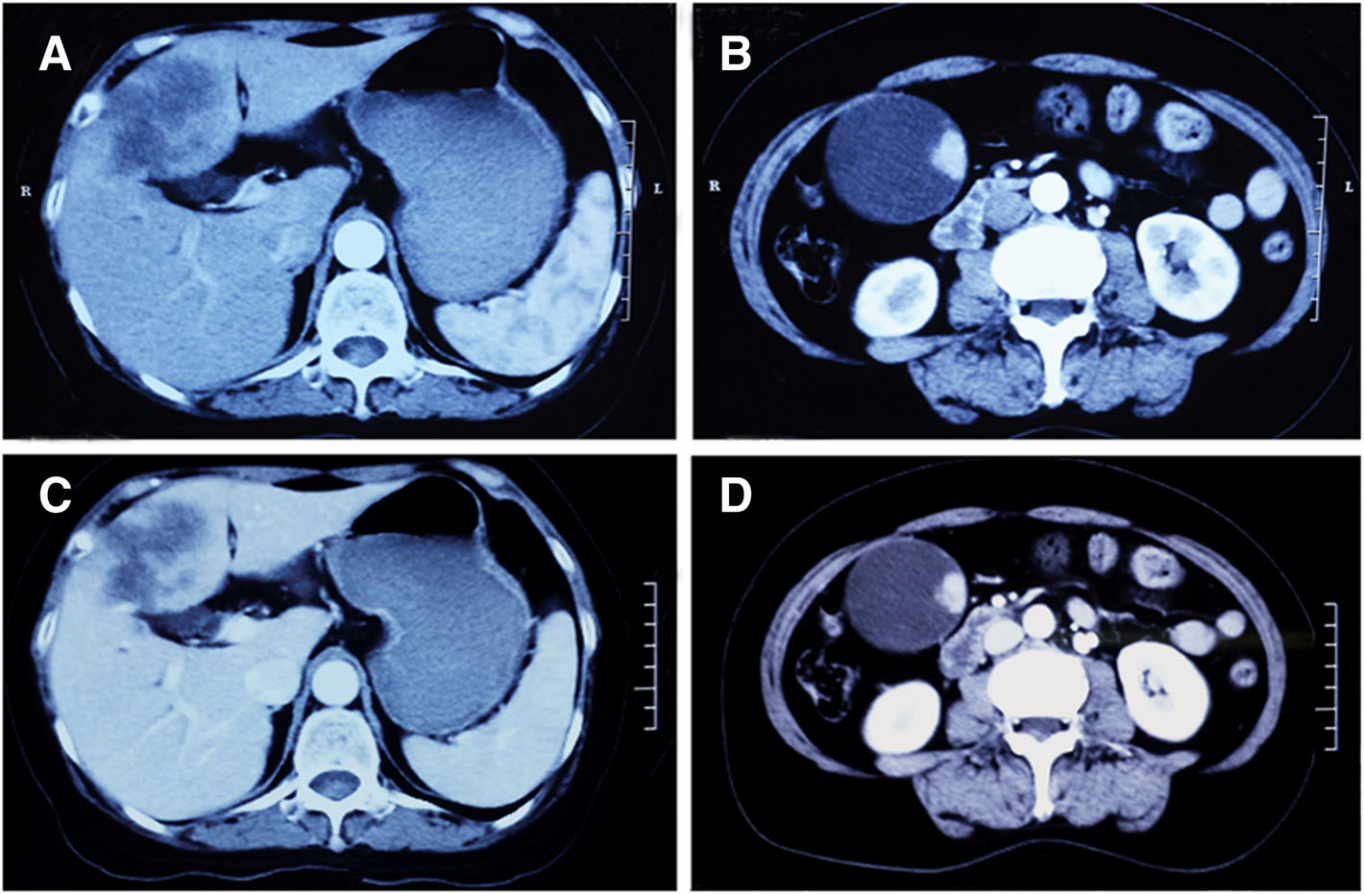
Abdominal multi-detector computed tomography-scan showing 3 × 3 cm mass in gallbladder and 6.2 × 4.5 cm lesion in segment IVb of the liver. a and b Artery phase, c and d Venous phase
